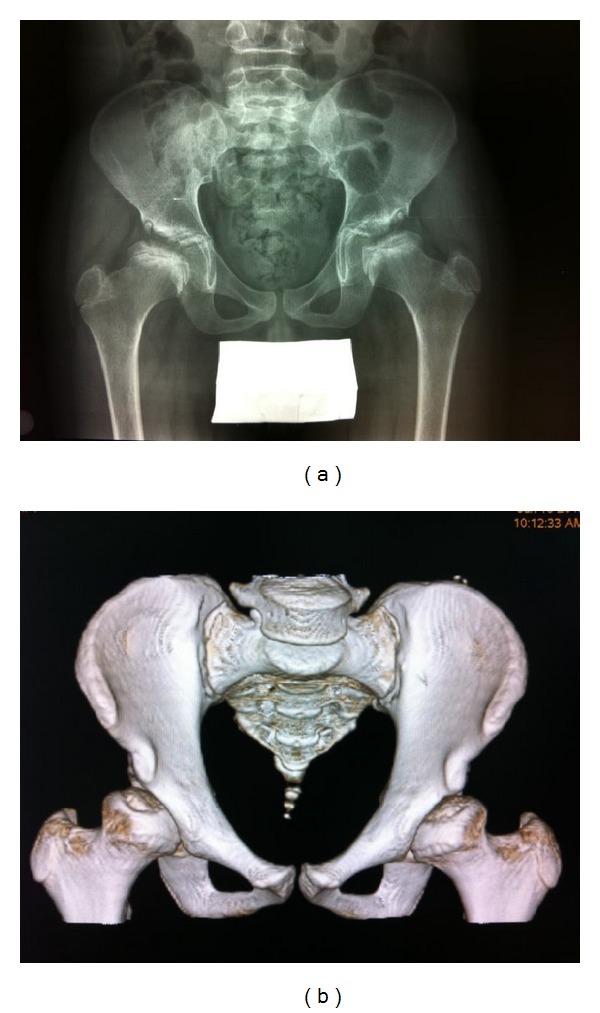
(a) First pelvic X-ray: dysplastic appearance of both femoral heads and irregular upper contour of the acetabular roof with mild coxa vara. (b) Last pelvic CT scan (three-dimensional volume-rendered reformatted picture): almost total reconstruction of anatomic structures.
